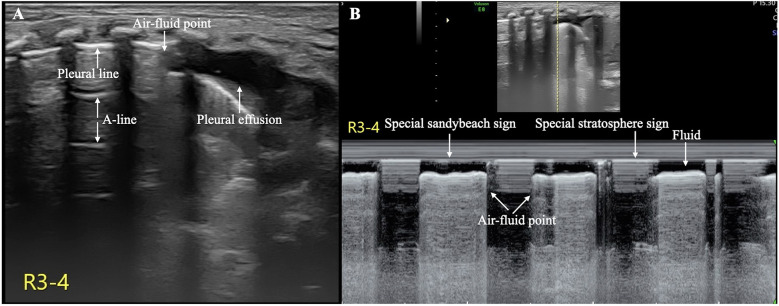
Hydropneumothorax in the right thoracic cavity. The pleural line and A-line are clearly displayed in the upper lung field, and an anechoic dark area is observed in the lower lung field on B-mode ultrasound (A) On real-time ultrasound, a demarcation point between the gas and fluid interfaces is observed; this kind of demarcation point is referred to as the air–fluid point in this paper (Supplementary Video S2). M-mode ultrasound revealed the air–fluid points as alternating points of the characteristic sand on the beach sign and the characteristic stratosphere sign (B) These findings confirmed that both pleural effusion and pneumothorax coexisted (i.e., hydropneumothorax).
